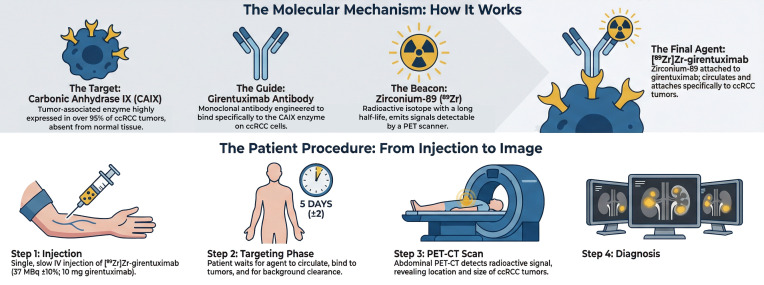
Graphical representation of the mechanism of girentuximab PET-CT, the procedure and its diagnostic performance. ccRCC = clear-cell renal cell carcinoma; CAIX = carbonic anhydrase IX; PET = positron emission tomography; IV = intravenous; PET-CT = positron emission tomography-computed tomography.
